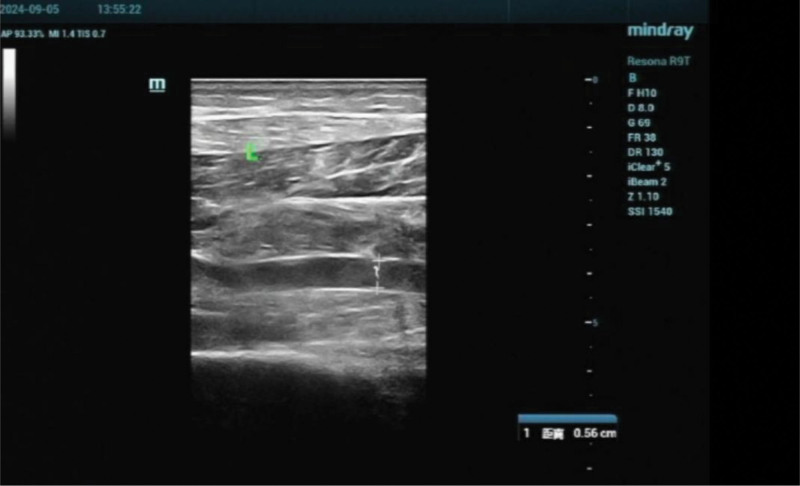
Doppler ultrasonography confirming thrombosis within the intermuscular vein of the left calf. AP = anteroposterior, DR = dynamic range, FR = frame rate, MI = mechanical index, SSI = spatial standard intensity, TIS = thermal index scan.
